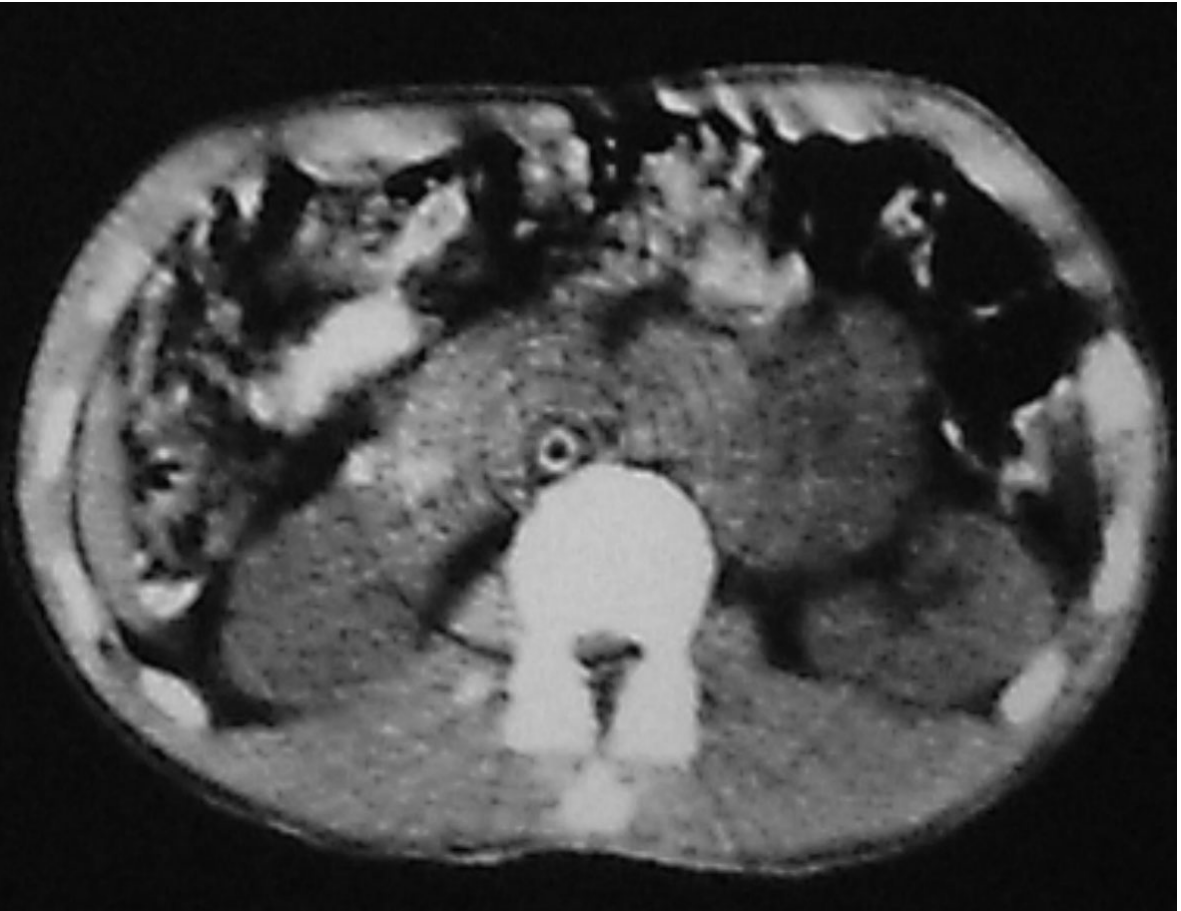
Computed tomography scan showing retroperitoneal residual mass after systemic chemotherapy in 1989.
